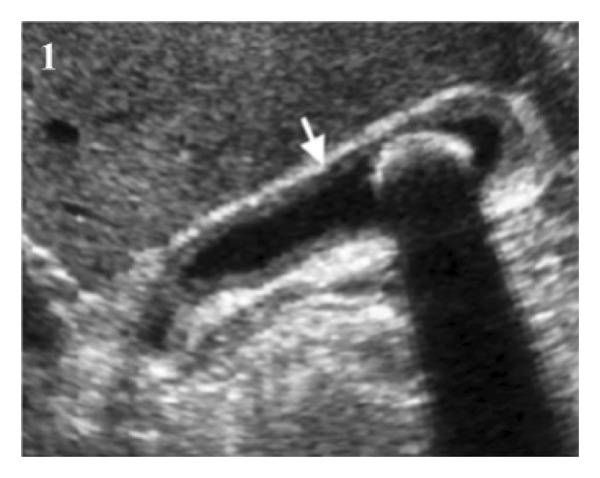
Ultrasound showing diffuse gallbladder wall thickening with stones.
